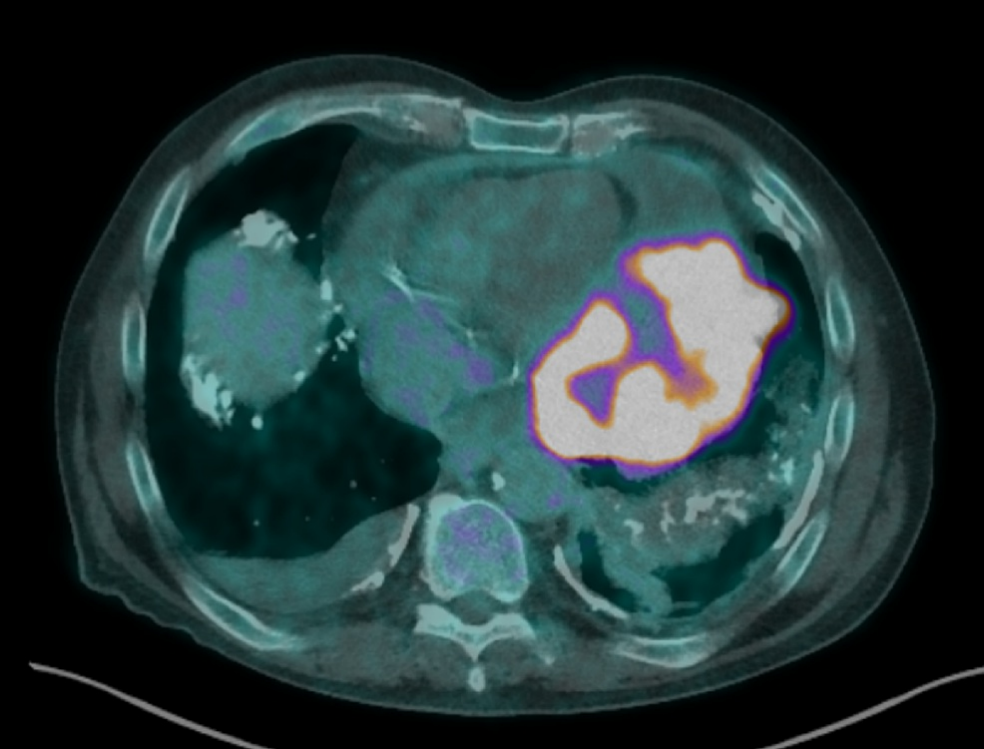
FDG-PET CT showing pericardial mass and effusion FDG: fluorodeoxyglucose; PET: positron emission tomography
